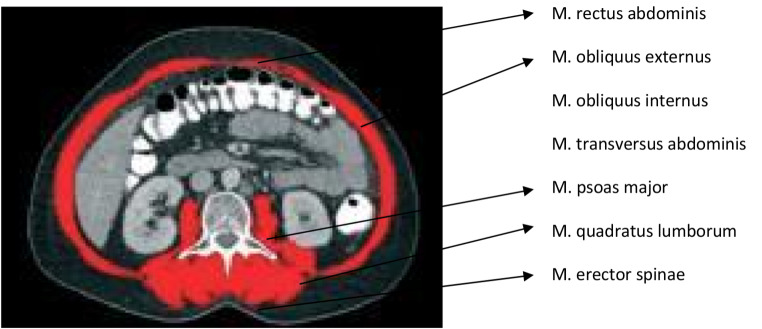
Total skeletal muscle area, Red painted (Abdominal CT, Axial Section, L3 level)
